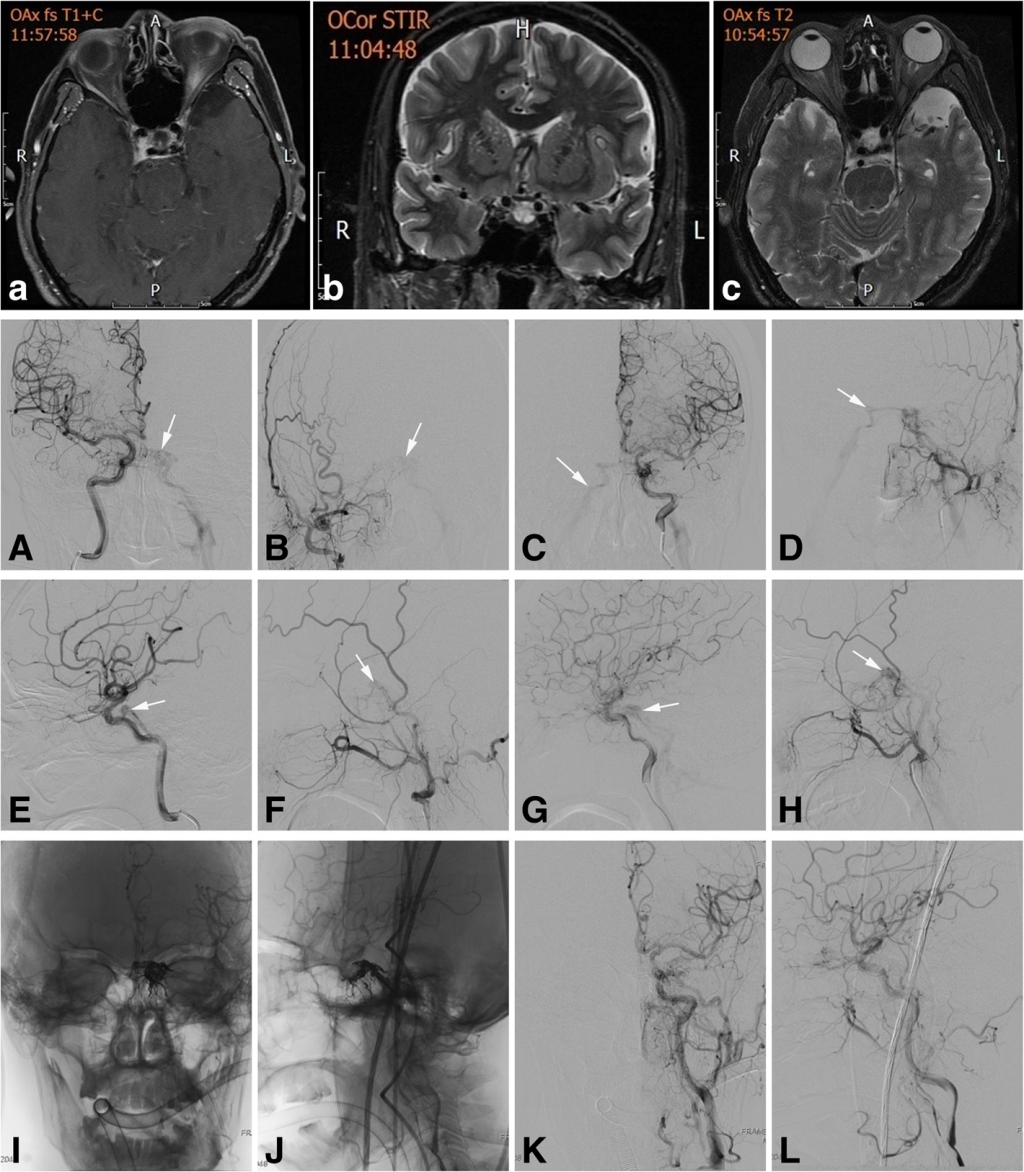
Enhanced T1-weighted (a) and T2-weighted (b,c) MRI showing normal cavernous sinuses. Early opacification of the bilateral cavernous sinuses: anteroposterior views of the right ICA (a) and ECA (b) and the left ICA (c) and ECA (d); lateral views of the right ICA (e) and ECA (f) and the left ICA (g) and ECA (h). Anteroposterior (i) and lateral (j) views showing the position of Onyx in the left cavernous sinus, the inferior petrosal sinus and the intercavernous sinus. Anteroposterior (k) and lateral (l) views of the left carotid confirmed complete occlusion of the CCF
